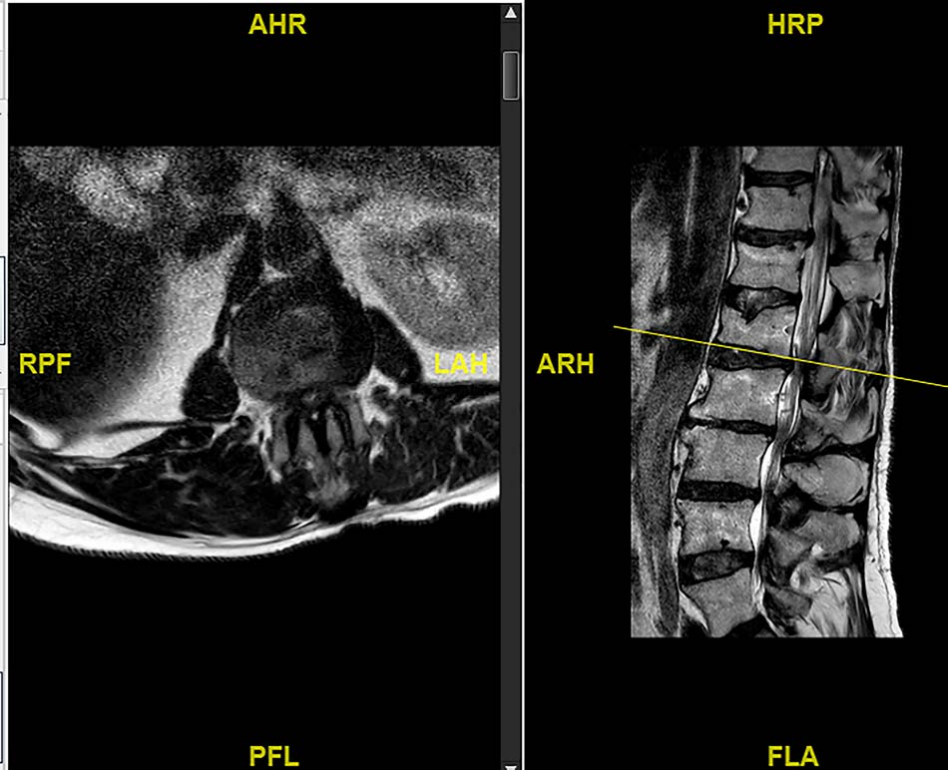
Initial MRI images prior to index procedure revealing stenosis at L1/2 and L2/3.
